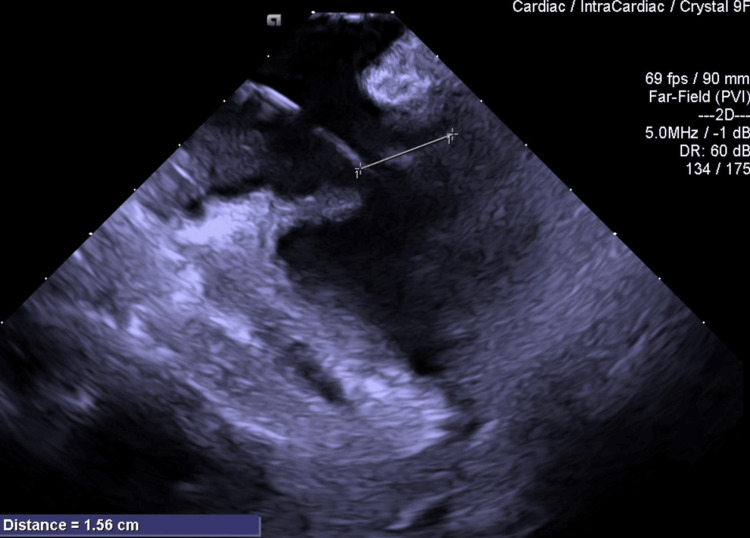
Measurement of patent foramen ovale (PFO) tunnel obtained using intracardiac echocardiography (ICE) Tunnel length measured to be 1.56cm, an elongated morphology associated with significant positional shunting and symptomatic platypnea-orthodeoxia syndrome (POS).
